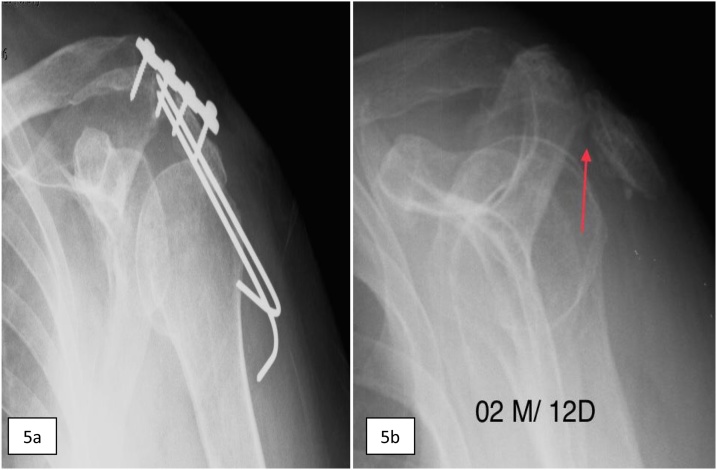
Post-operative left shoulder x-rays. a) AP view (immediate P.O.); b) outlet view, red arrow: acromion fracture after removal of osteosynthesis material.
